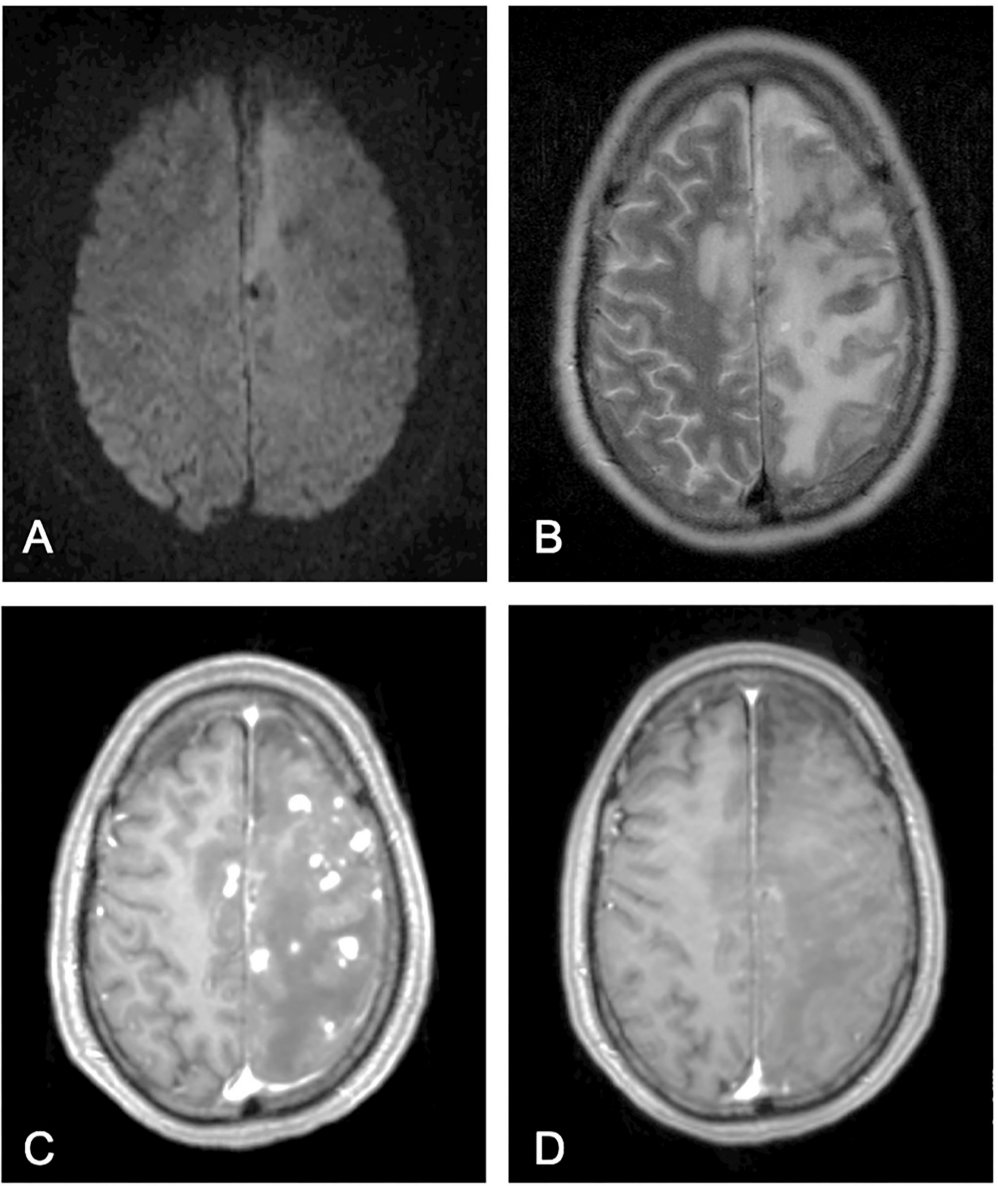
Magnetic resonance imaging. Magnetic resonance imaging (MRI) findings at time of re-admission 6 weeks after initial discharge [(A) Diffusion-weighted axial imaging, (B) T2 weighted axial imaging and (C) contrast enhanced T1 axial imaging] and 10 days later after corticosteroid treatment [(D) contrast enhanced T1 axial imaging].
